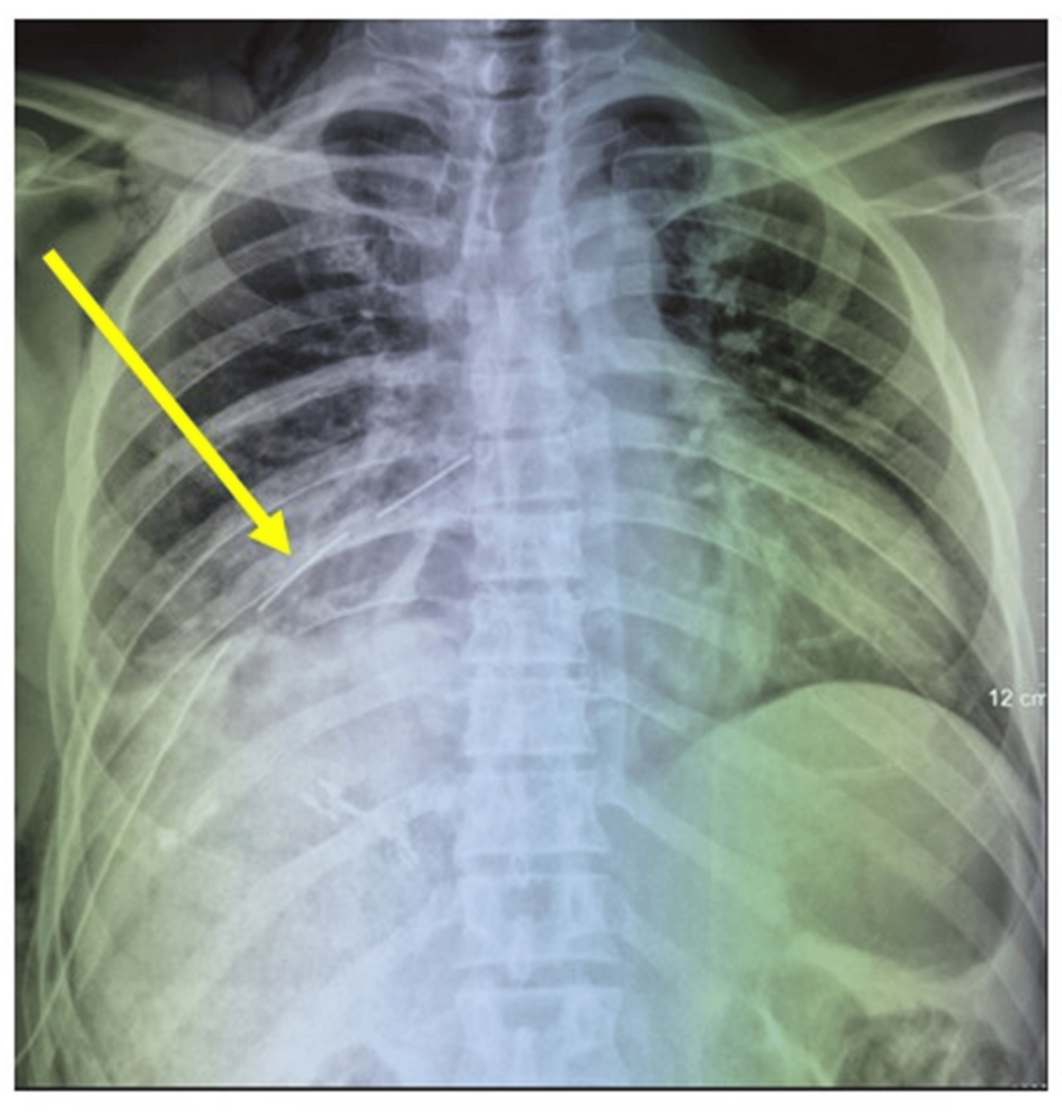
Chest X-ray revealed a lung adherent to the wall, with a drain in place (yellow arrow) and disappearance of mediastinal opacity.
